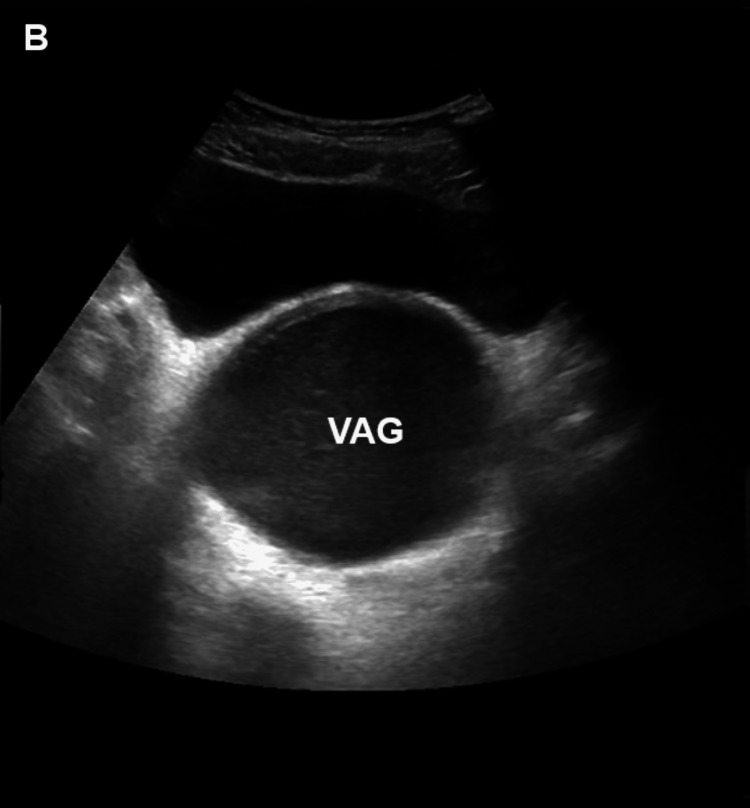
Ultrasound showing a distended vaginal canal with hypoechoic material
